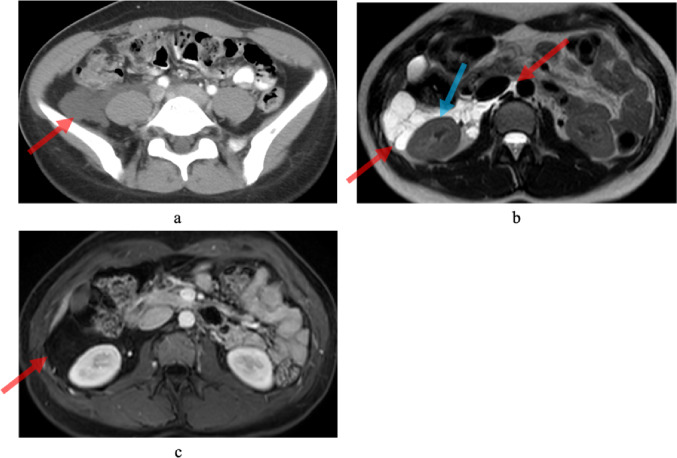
Retroperitoneal Lymphangioma. An axial contrast-enhanced CT image (a) shows a lobulated low-density mass within the right inferior retroperitoneum (red arrows). An axial T2-weighted image (b) shows a multi-septated cystic right retroperitoneal mass (red arrows) insinuating around the right kidney (blue arrow). The mass is non-enhancing on an axial fat-saturated T1-weighted contrast enhanced image (c)
